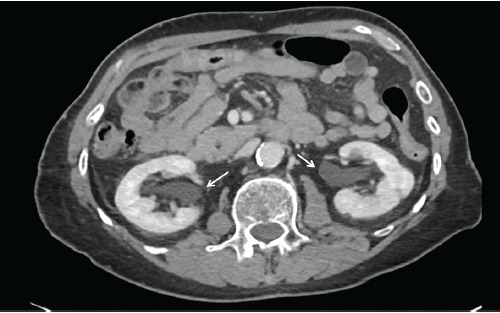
Axial computed tomography with intravenous contrast at the level of the kidneys shows mild hydronephrosis and hydroureteronephrosis from bladder outlet obstruction (white arrows).
